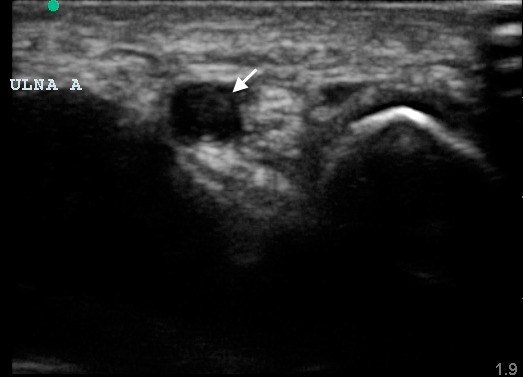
Ultrasound image of the distal ulnar artery in transverse plane noting echogenic thrombus (arrow) within the vessel lumen.
